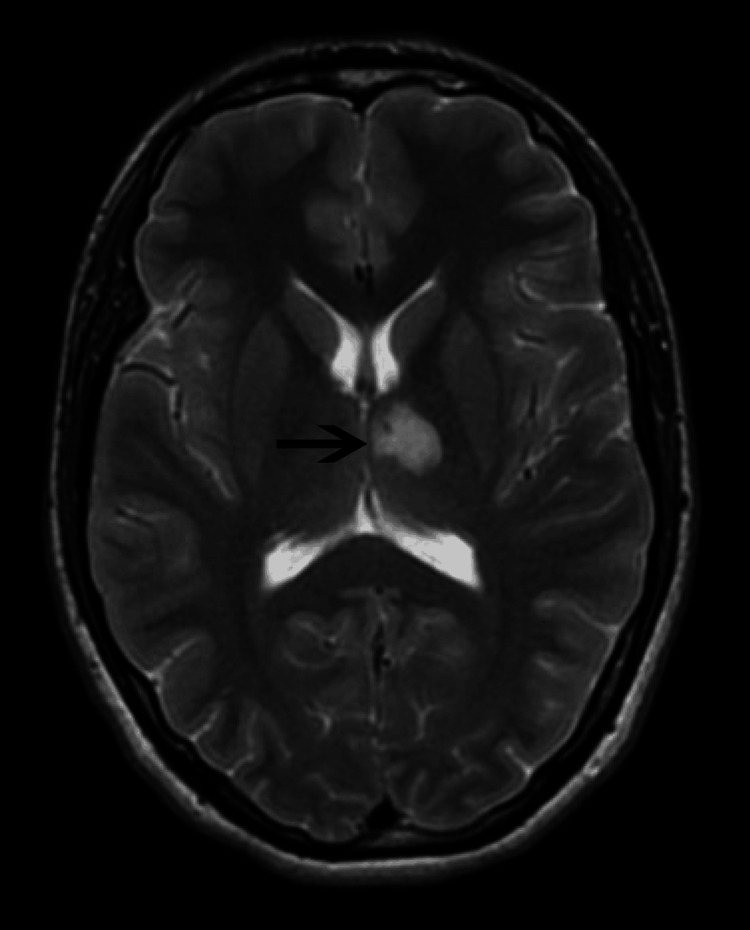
MRI of the brain showing an acute left thalamic infarct
